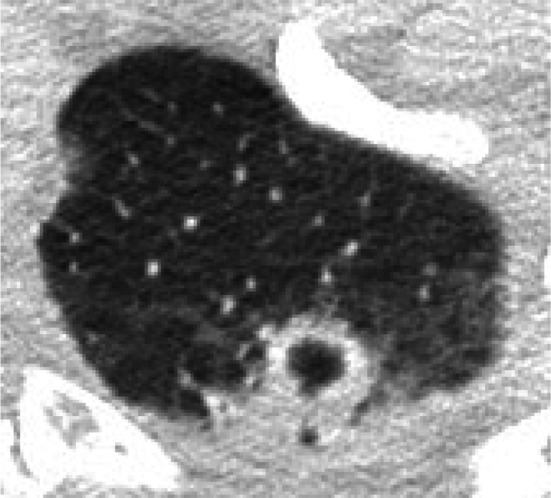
Another computed tomography scan performed 2 days before living-donor liver transplantation. A small nodular-shaped halo sign was observed
